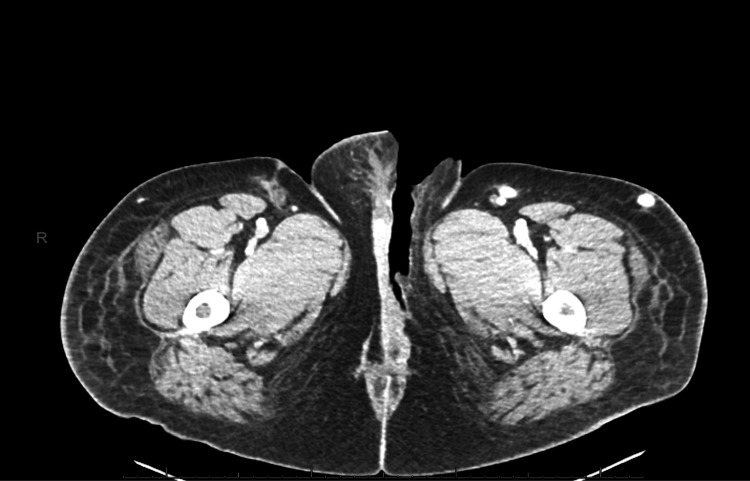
Post-surgical contrast CT of the pelvis Post-surgical changes of the labial tissue with no obvious aggressive new cross-fascial inflammatory extension or drainable fluid collection
